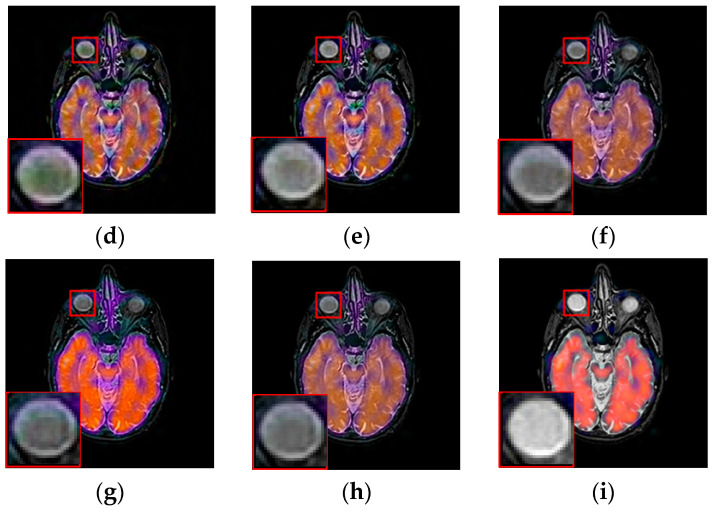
Fused results of MRI/SPECT medical images by different methods. (a) MRI; (b) SPECT; (c) DCTWT; (d) Curvelet; (e) NSCT; (f) Liu-ASR; (g) Kim; (h) Zhu; (i) Proposed.
